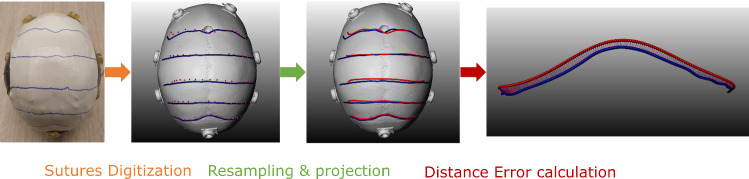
Followed steps for digitization of the drawn sutures and the calculation of distance error between drawn (red) and CT-annotated (blue) sutures
